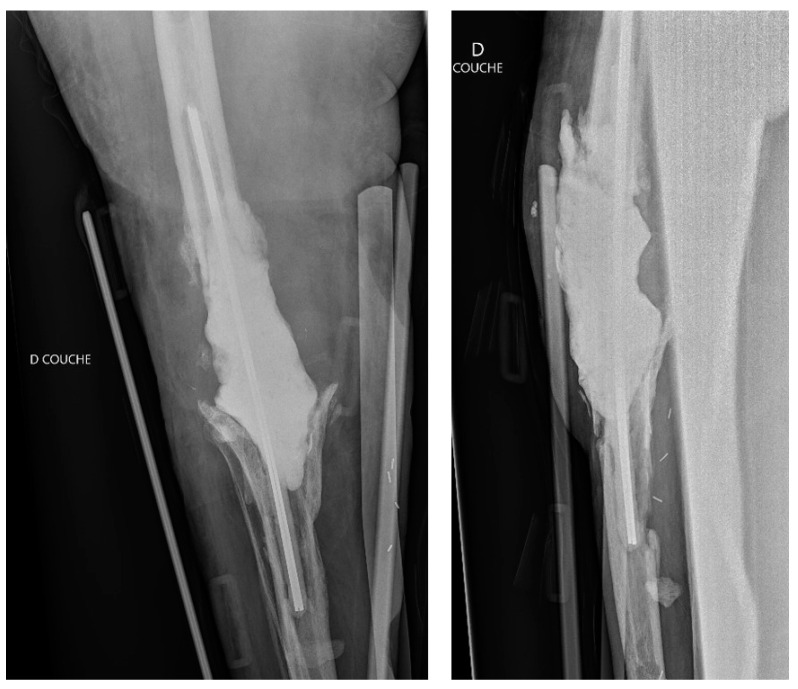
Anteroposterior and lateral post-operative radiographic views of the right knee after component explanation with an antibiotic-loaded cemented spacer, fixed with two metalic rods.
